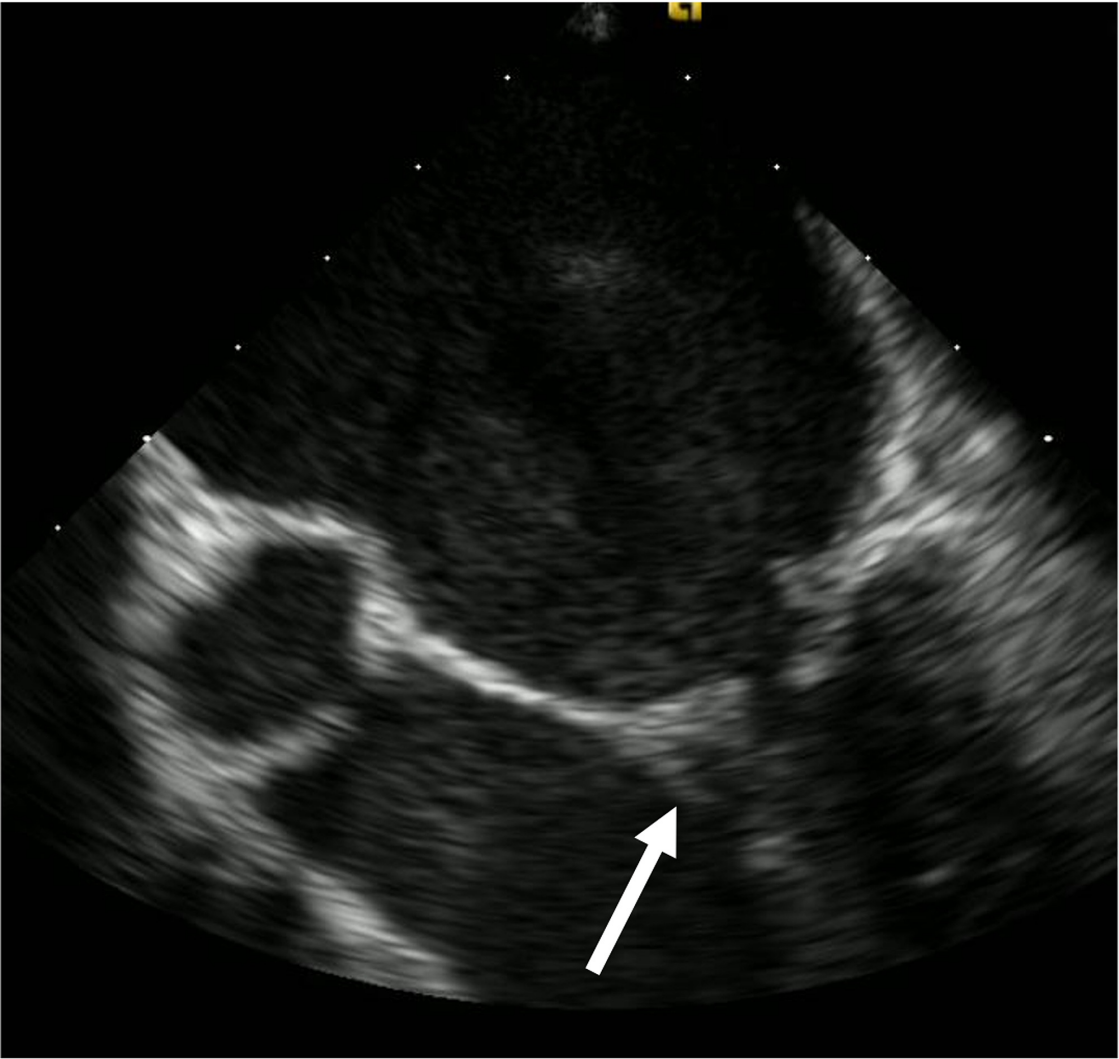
Transesophageal echocardiogram demonstrating a small independently mobile linear echo-density on the posterior leaflet of the mitral valve (white arrow), consistent with a vegetation.
